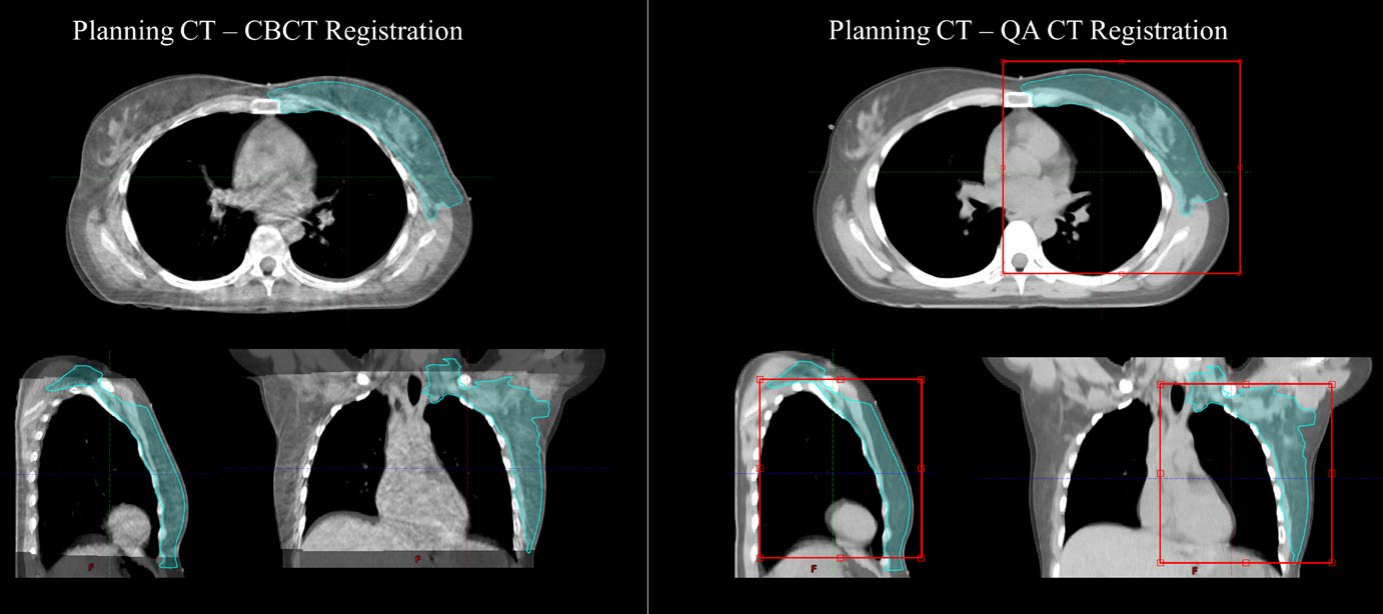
Cone‐beam computed tomography (CBCT) setup registration. Left: Orthogonal views of the planning CT and CBCT fusion. Right: Orthogonal views of the planning CT and quality assurance CT (QA‐CT) fusion with the volume used for image registration indicated by the red box. This registration volume was restricted to be within the CBCT field of view and focused on the treated volume
